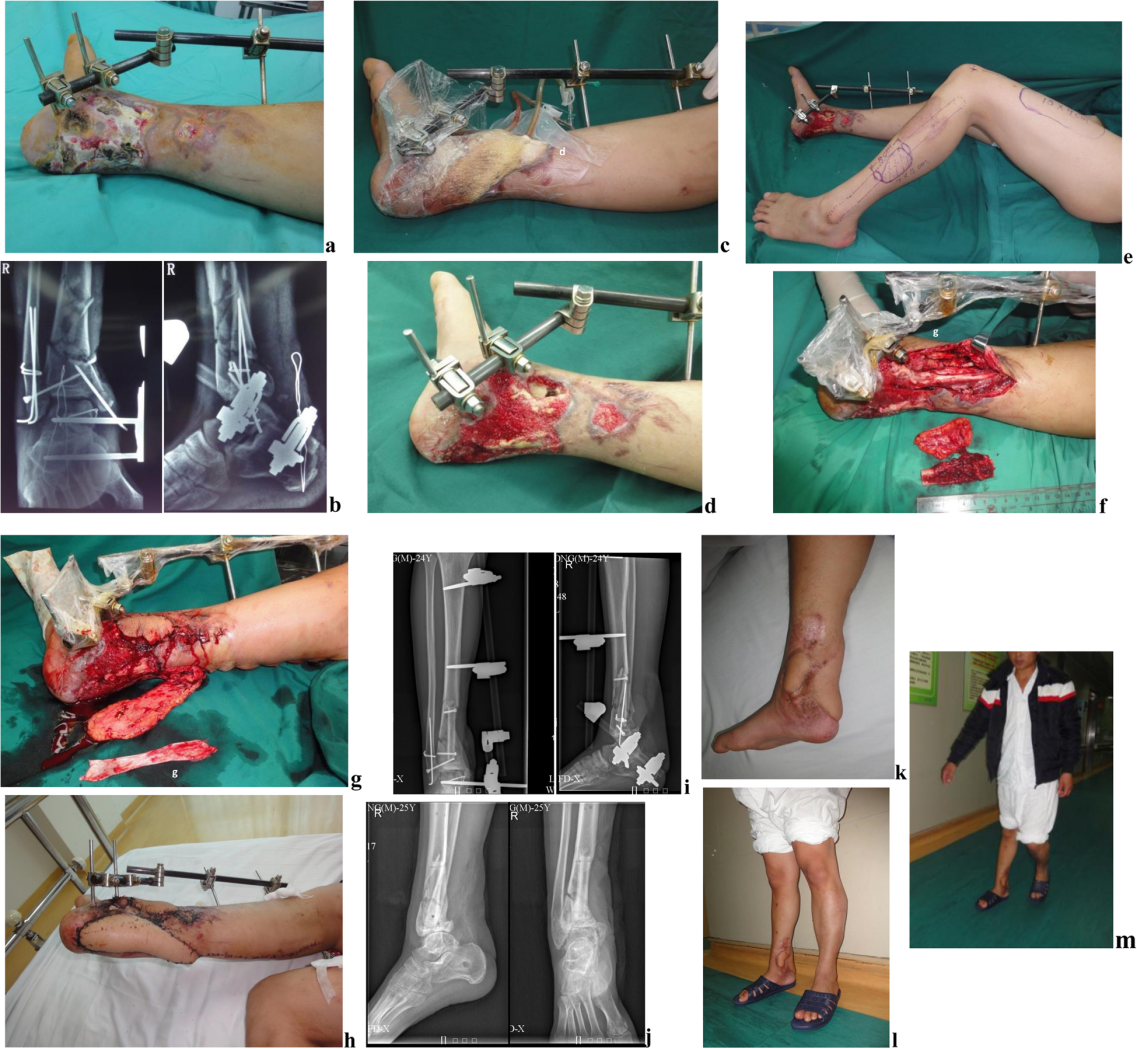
Case 1. A 24-year-old male patient with multiple fractures of the distal tibia and fibula complicated with soft tissue defects and infection for more than 1 month. Injury was caused by a traffic accident. Bone injury appearance upon admission and X-ray of the ankle joint (a, b). After thorough debridement and vacuum sealing drainage (VSD) of the right ankle, the granulation tissues on the wound surface grew well (c, d). During the second-stage procedures, FVFG was performed to reconstruct the infected bone defects (approximately 6 cm in size) at the distal tibia. The fascia lata of the same thigh was designed to repair the Achilles tendon defects. Simultaneously, the sural neurovascular flap of the right limb was reversely transferred to repair the Achilles tendon wound. The contralateral fibular bone flap, thigh fascia lata, and the ipsilateral sural neurovascular flap were harvested (e–g). Both the fibular flap and sural neurocutaneous flap survived well, and the wound was healed without exudation after operation (h). Postoperative X-ray showed that the FVFG repaired the distal tibial defects with excellent alignment (i). External fixator was removed 6 months postoperative and partial weight-bearing walk under the protection of the brace. At 1 year after operation, the internal fixator was removed, and normal walking function was restored. (j–m). After 24 months of postoperative time, the ASAMI functional score of the affected extremity was excellent, with an external fixation index 1.0
